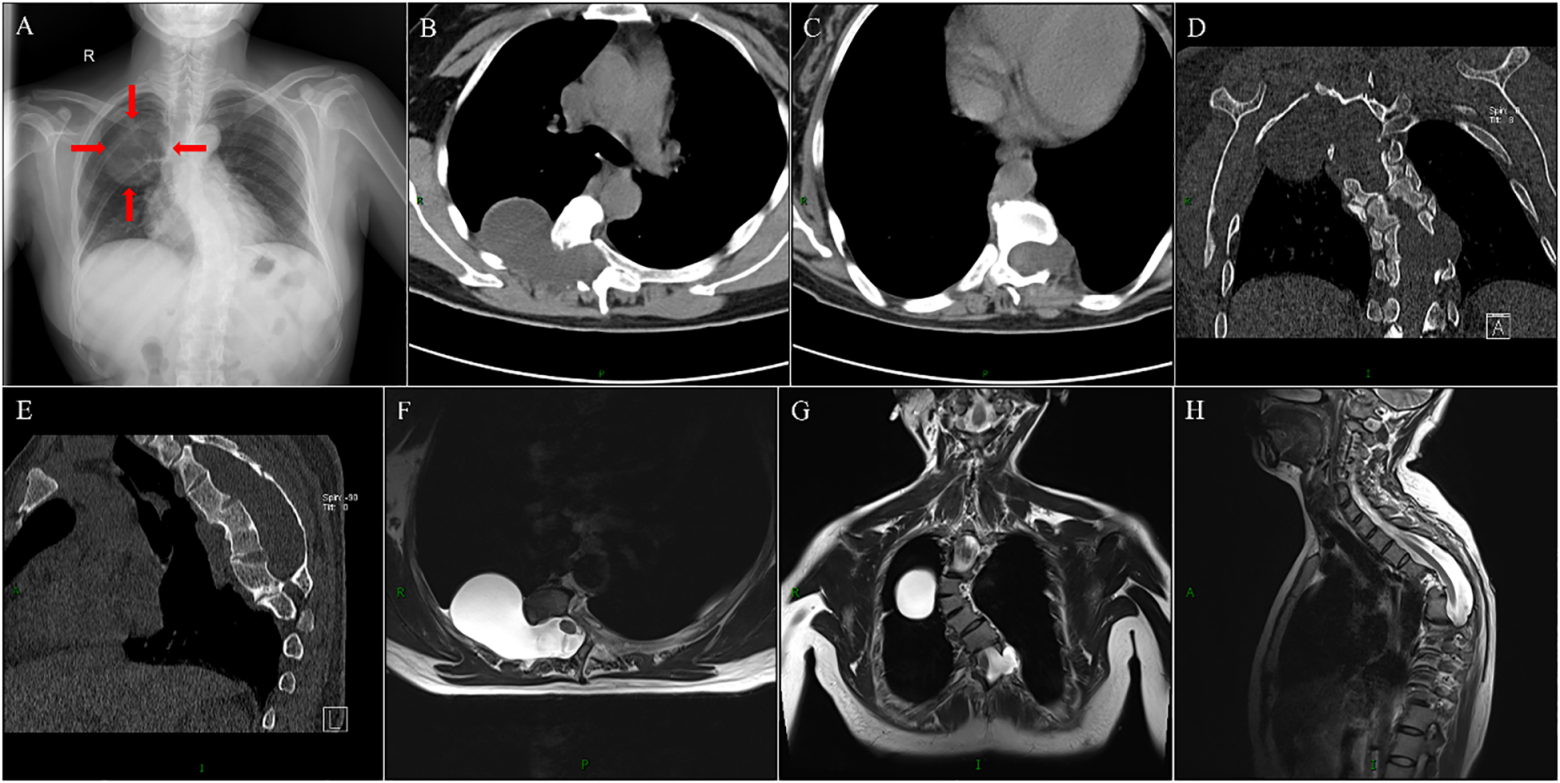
Imaging findings from April 2015. Chest X-ray demonstrates an “S”-shaped scoliosis deformity and a space-occupying lesion (later confirmed as meningocele) in the right upper-middle lung field (A). Thoracic spine CT reveals enlargement of the right T5/T6 and left T9/T10 intervertebral foramina, with meningoceles protruding into the thoracic cavity (B-E). Additionally, thoracic spine CT shows fusion deformity of T4-T9 vertebral bodies, vertebral body bone thinned due to compression, and spinal canal enlargement (B-E). On thoracic spine MRI T2-weighted images (T2WI), meningoceles within the right T5/T6 and left T9/T10 intervertebral foramina appear homogeneously hyperintense with clear boundaries, communicating with the dilated thecal sac (F-H).
